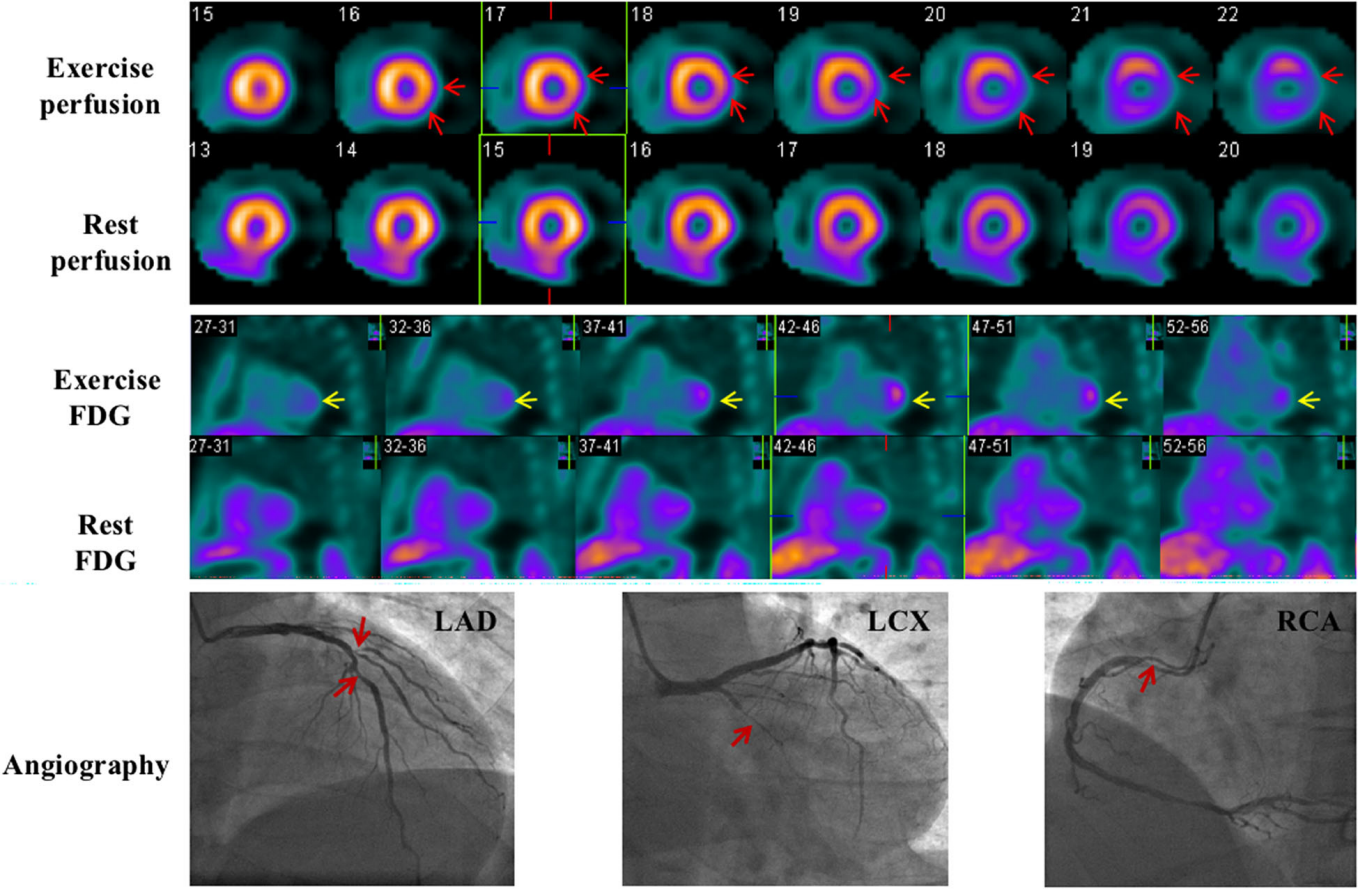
Images of a 39-year-old man. He had a 70% stenosis in the LAD and a 90% stenosis in the diagonal branch, a 100% stenosis in the LCX, and a 90% stenosis in the proximal RCA. Perfusion images show reversible defects in the lateral wall (*red arrows*). Resting ^18^F-FDG images indicate background uptake in the cardiac cavities and no visible uptake in the left ventricular wall (‘none’ pattern), exercise ^18^F-FDG images exhibit intense uptake in the lateral wall (‘focal’ pattern, *yellow arrows*) which is in accordance with exercise perfusion images
